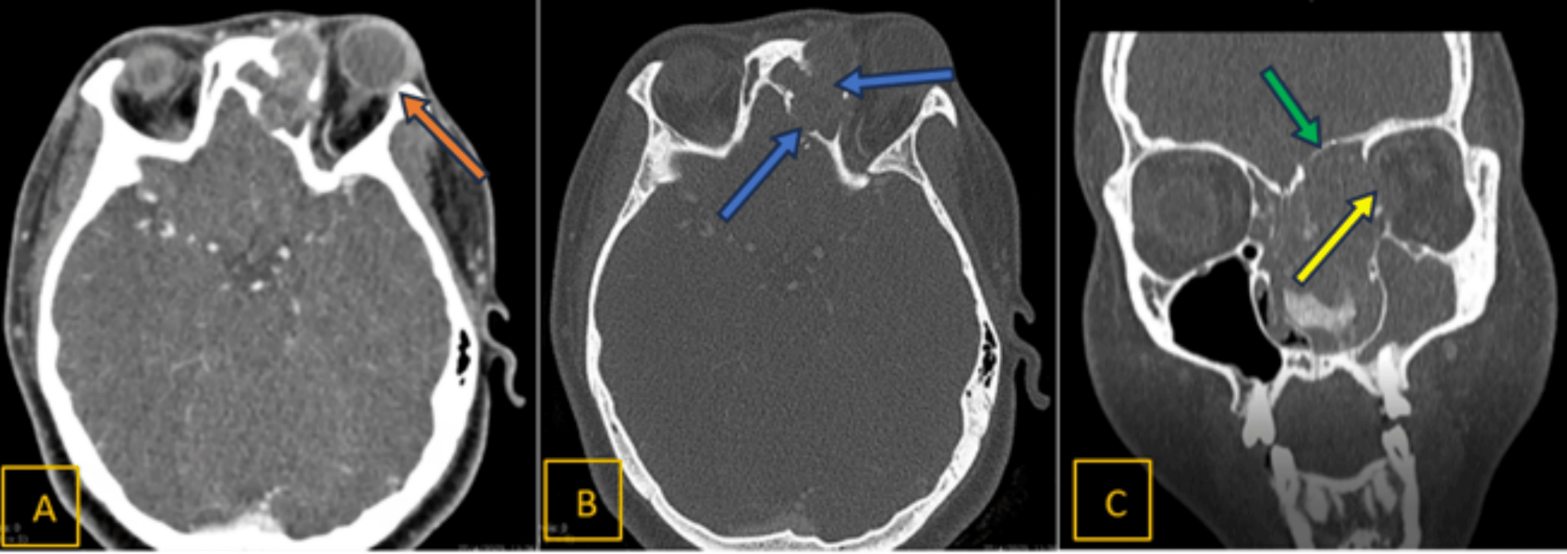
CT with contrast: different views (A, B) Axial view. (C) Coronal view. Orange arrow: mild ptosis of the left eye. Blue arrow: the anterior and posterior walls of the frontal sinus are eroded by the mass. Yellow arrow: eroded lamina papyracea. Green arrow: erosion of the superomedial wall of the left frontal sinus CT: computed tomography
